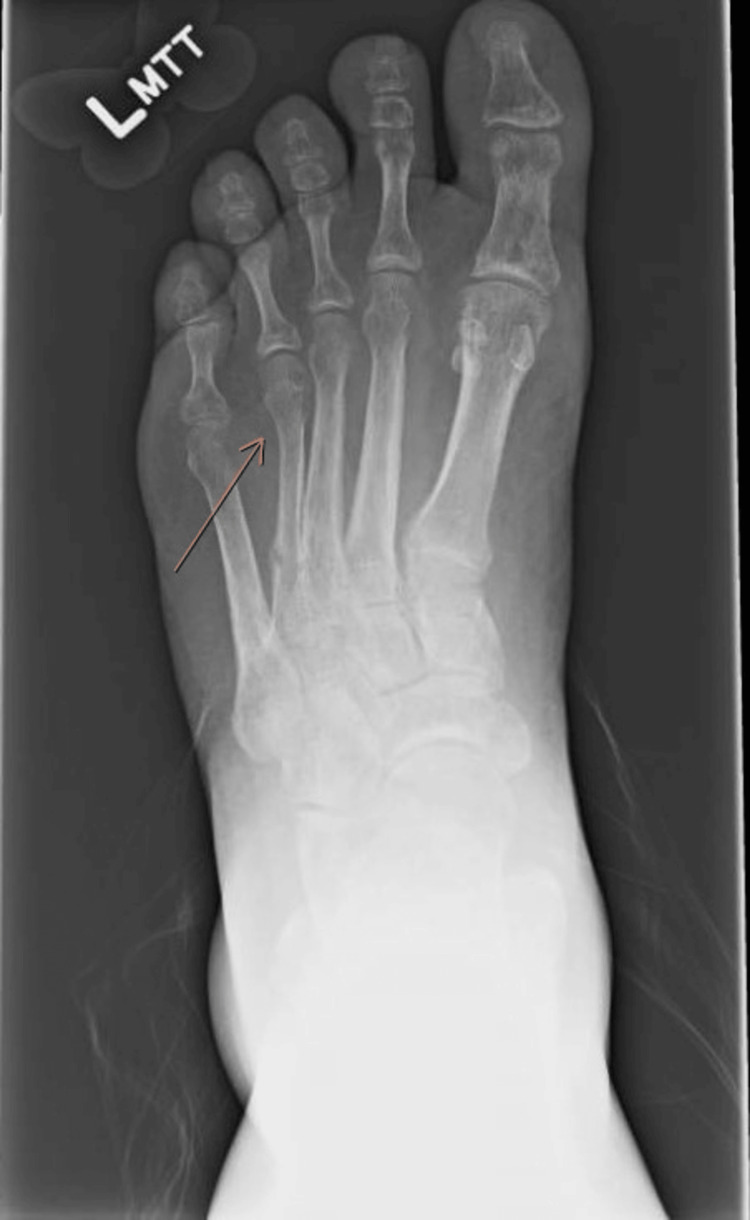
Radiograph of the left foot Demonstrates healing of a proximal diaphyseal fracture of the fourth metatarsal with interval healing, along with inferior and posterior calcaneal spurring, and generalized osteopenia.
